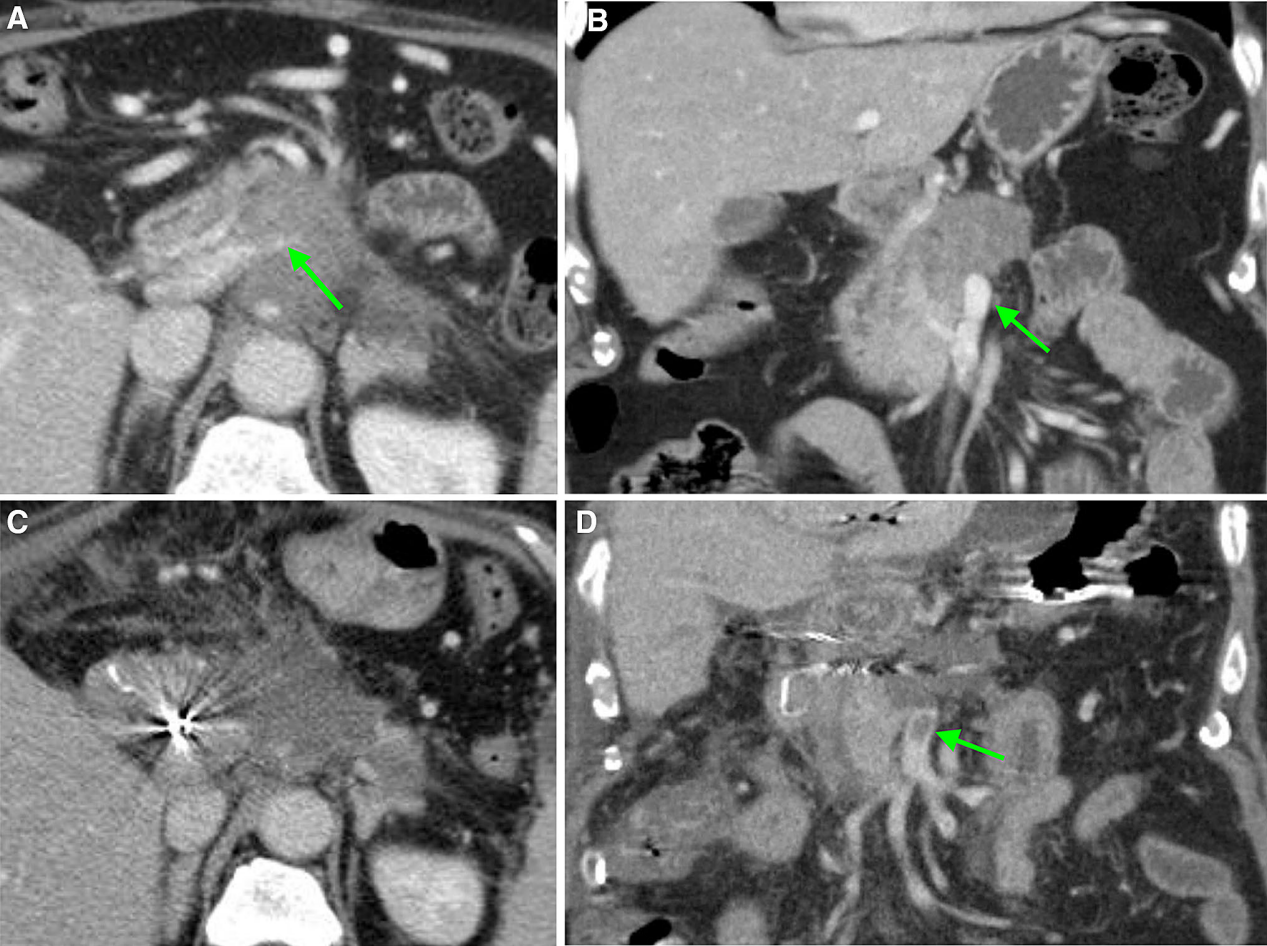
CT-images in the coronal plane, of a 66-year-old woman with a ductal adenocarcinoma in the head, neck and corpus of the pancreas. A The CT-image pre-RFA, shows a superior mesenteric vein (SMV) circumferential involvement of > 270°, with a pre-existent lumen reduction of > 50% (arrow) and B no thrombus present (arrow). C On the CT-image 1-week post-RFA the SMV and portal vein are occluded within the ablation zone and D a local thrombus is present in the SMV (arrow)
